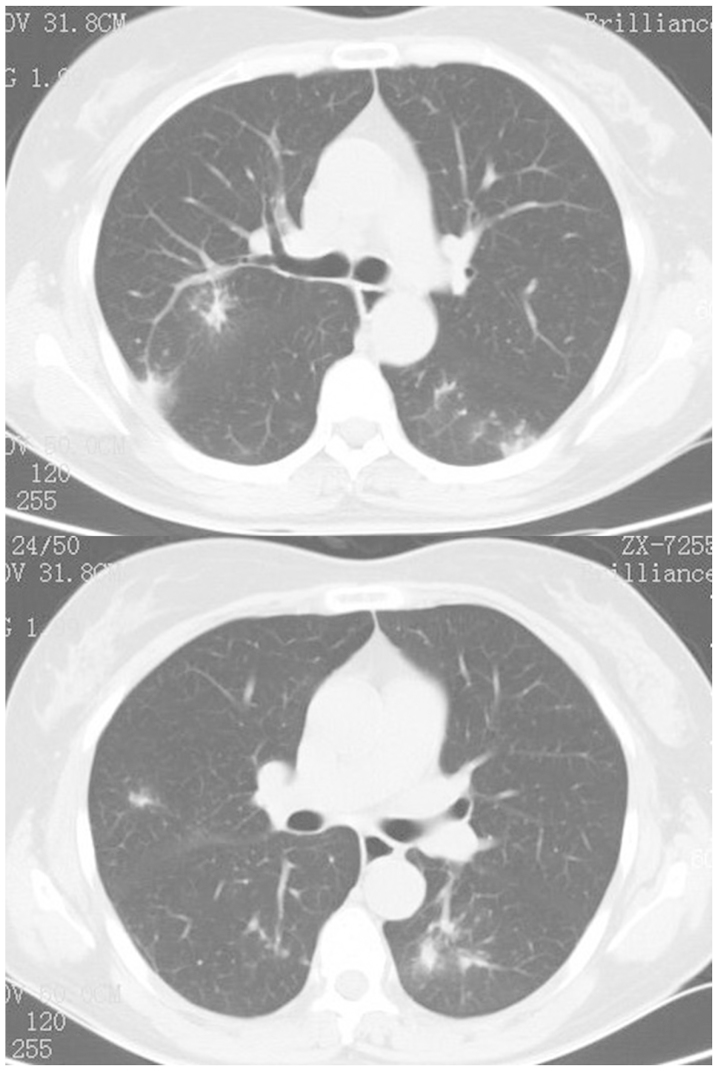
Chest radiography ten days after treatment showing patchy, nodular consolidation in the right lung and the lower lobe of the left lung.
